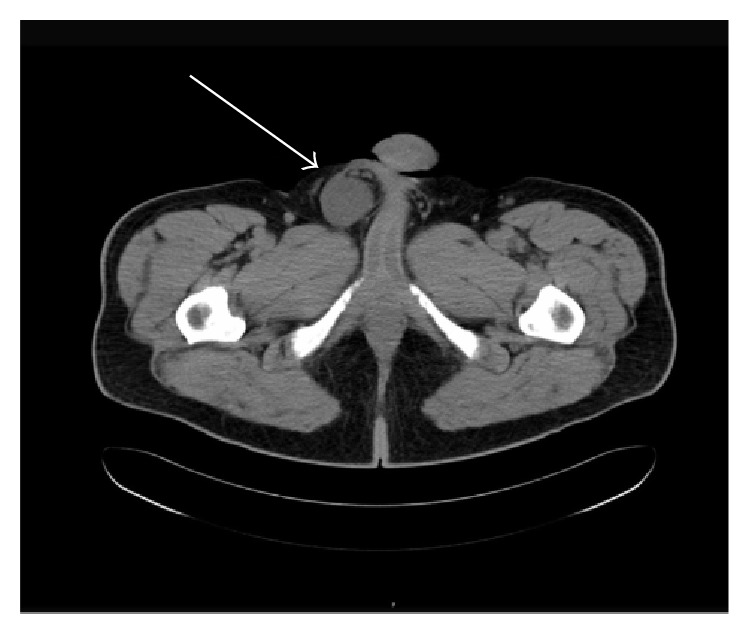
CT scan showing the solid soft-tissue paratesticular mass in contact with the right spermatic cord (white arrow).
